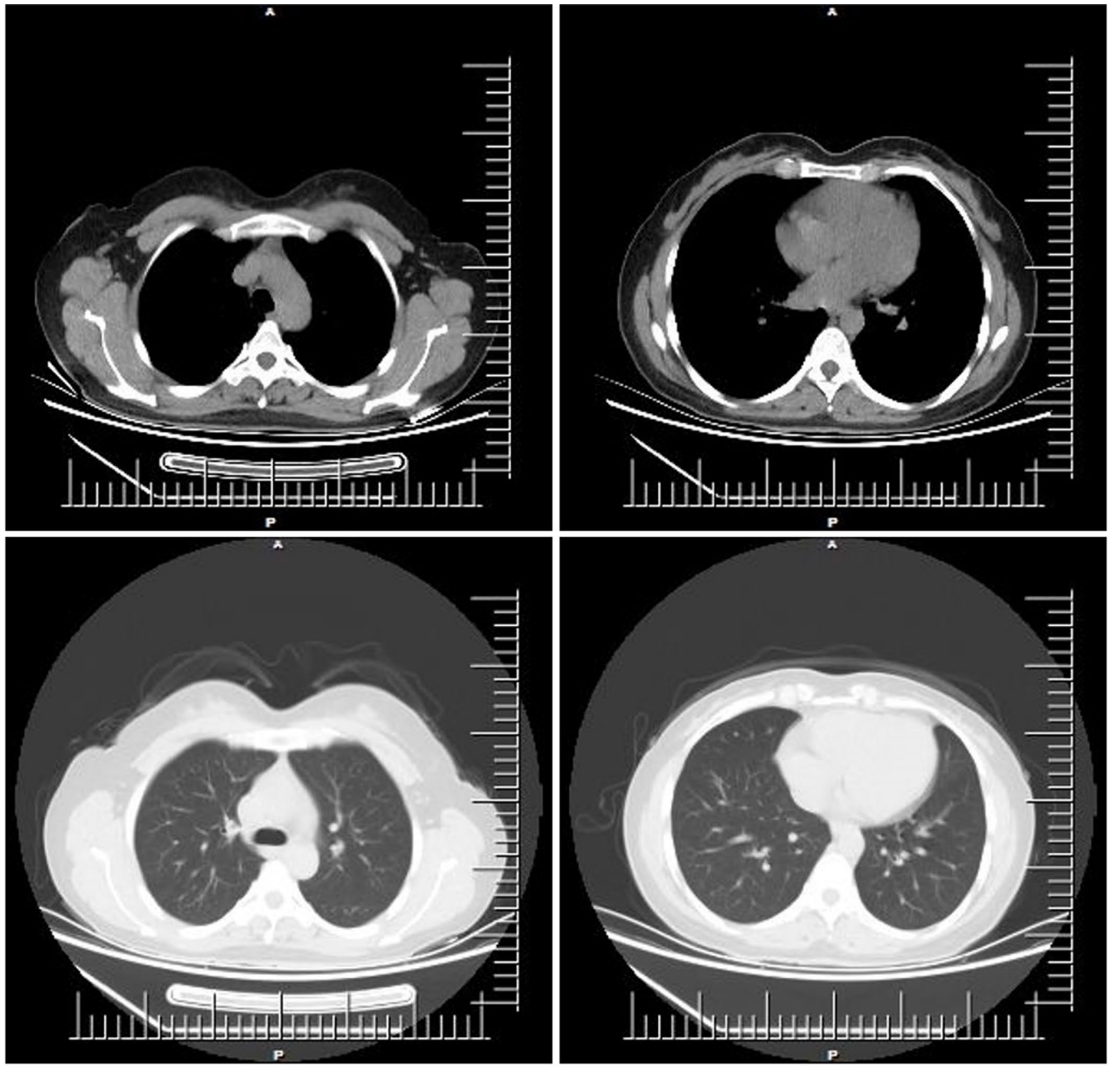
The representative chest computed tomography (CT) images of the patient.
